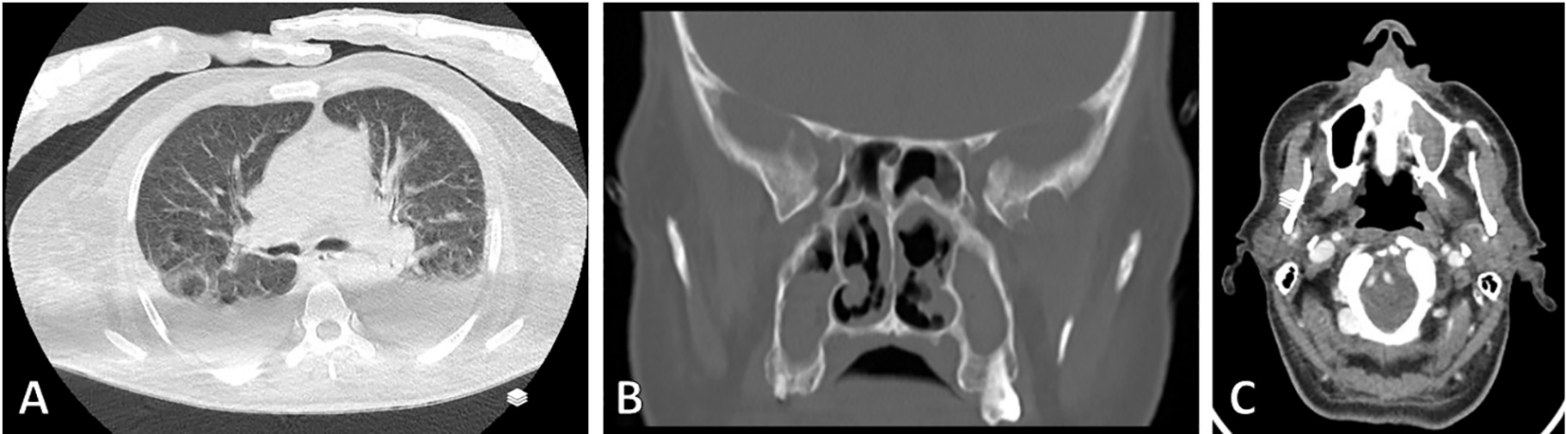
(A) Chest computed tomography scan demonstrating atelectasis in the right lower lobe, patchy consolidation in the left upper lobe, bilateral patchy ground-glass opacity, bilateral pleural effusion, pericardial effusion, right collapse consolidation, and air bronchogram. (B, C) Paranasal CT scan showing bilateral medial and sphenoid opacification, ethmoid destruction, medial septum destruction in the left maxilla, and bilateral frontal mucosal thickening.
